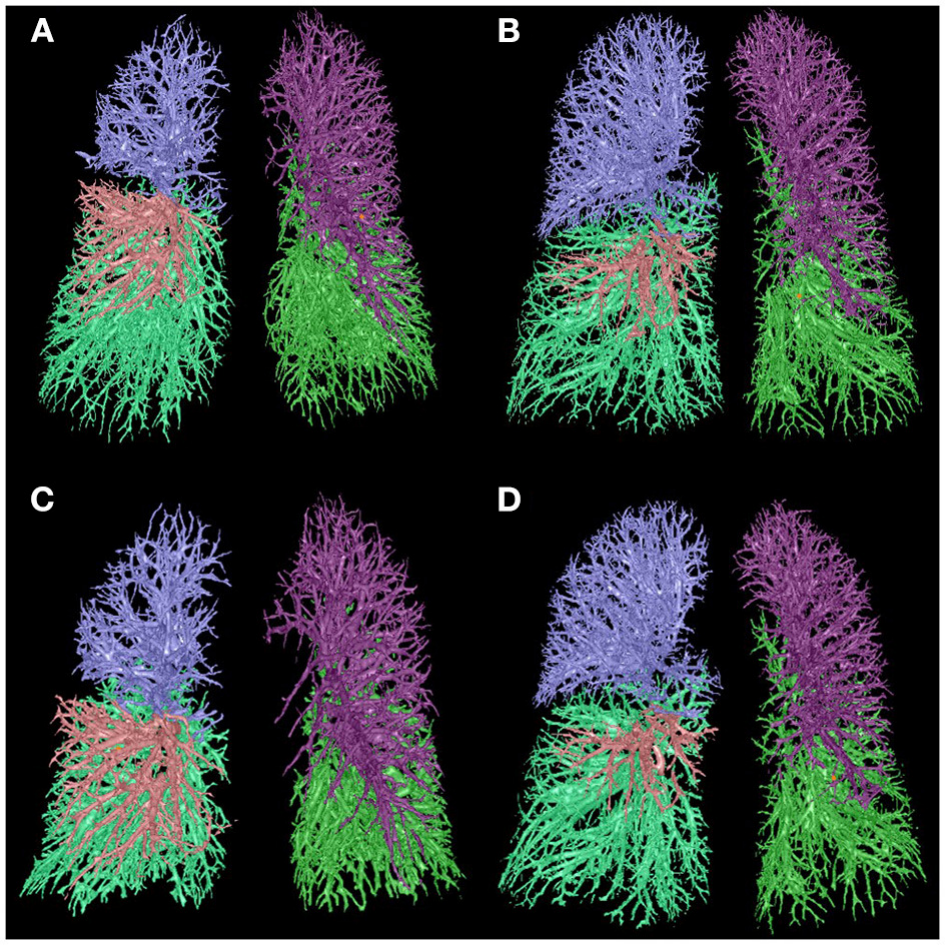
Comparison of IPVV between mild and severe COPD in inspiratory and expiratory CT. (A,C) A male with mild COPD (GOLD II, 68 years): a, the IPVV of the whole lung in inspiratory CT is 209.95 ml; b, the IPVV of the whole lung in expiratory CT is 169.10 ml. (B,D) A male with severe COPD (GOLD III, 64 years): a, the IPVV of the whole lung in inspiratory CT is 208.31 ml; b, the IPVV of the whole lung in expiratory CT is 201.18 ml.
